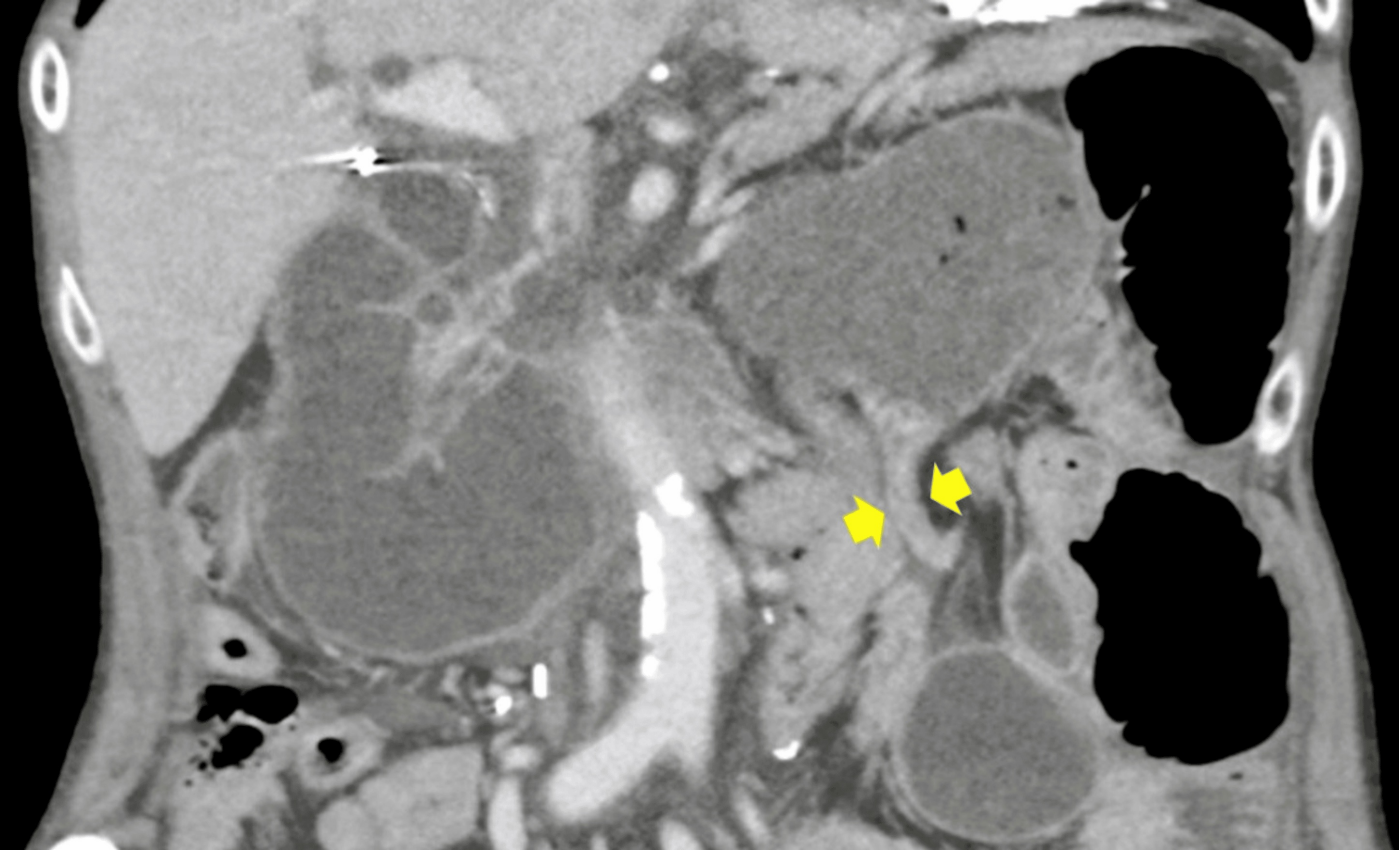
Transition Point Causing Obstruction of the Colonic Conduit Contrast-enhanced thoracoabdominal CT showing marked distension of the alimentary limb, with a transition point identified (yellow arrows), likely related to an adhesion in the context of the patient’s prior surgical history.
